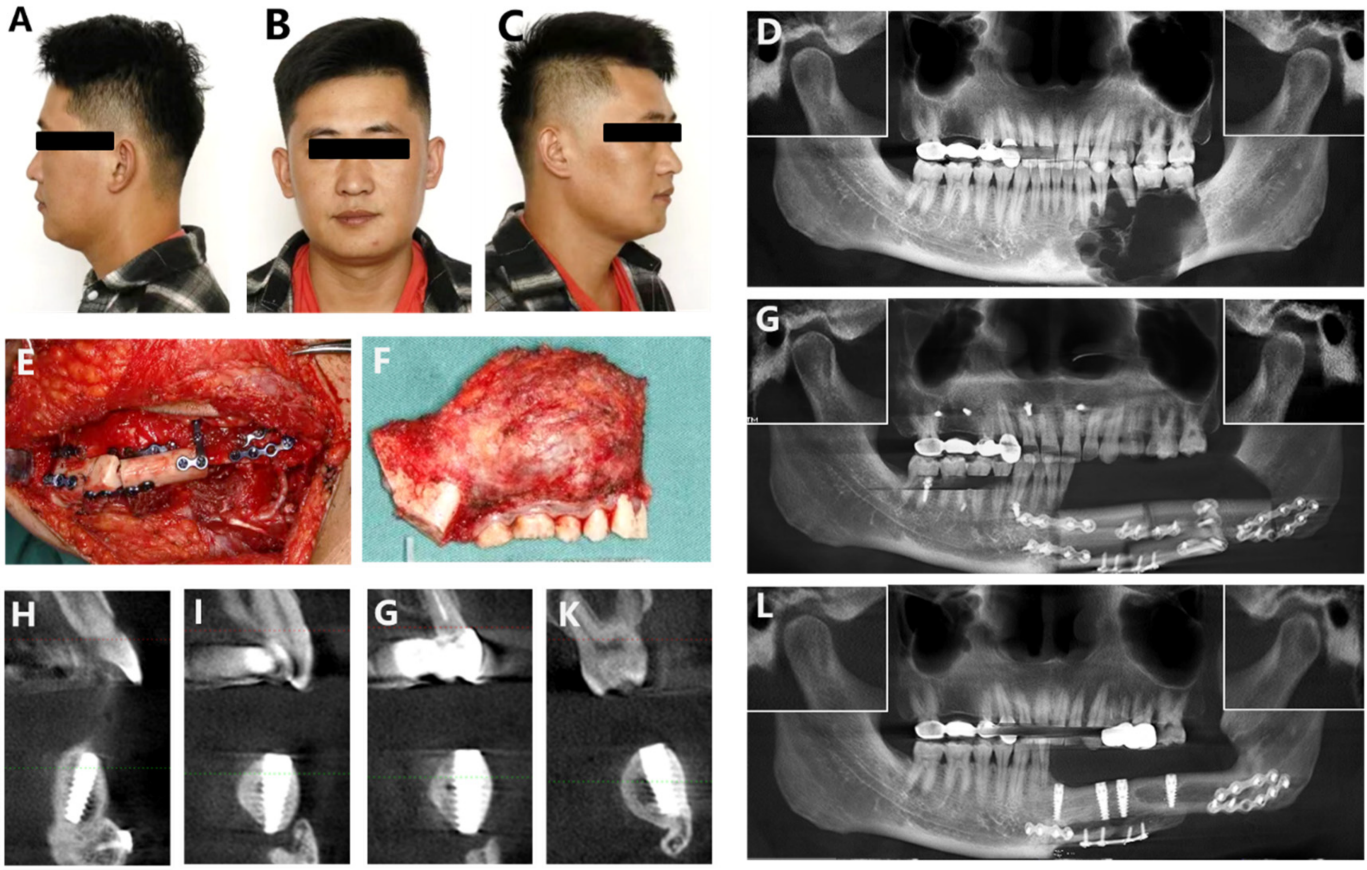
(A–C) Facial appearance before treatment, including front and side views. (D) Ameloblastoma preoperative CBCT. (E) Peroneal muscle flap transfer repair intraoperative. (F) Resection of the diseased mandibular segment. (G) Ameloblastoma postoperative CBCT. (H–K) Implant buccal and lingual imaging. (L) CBCT after implantation.
